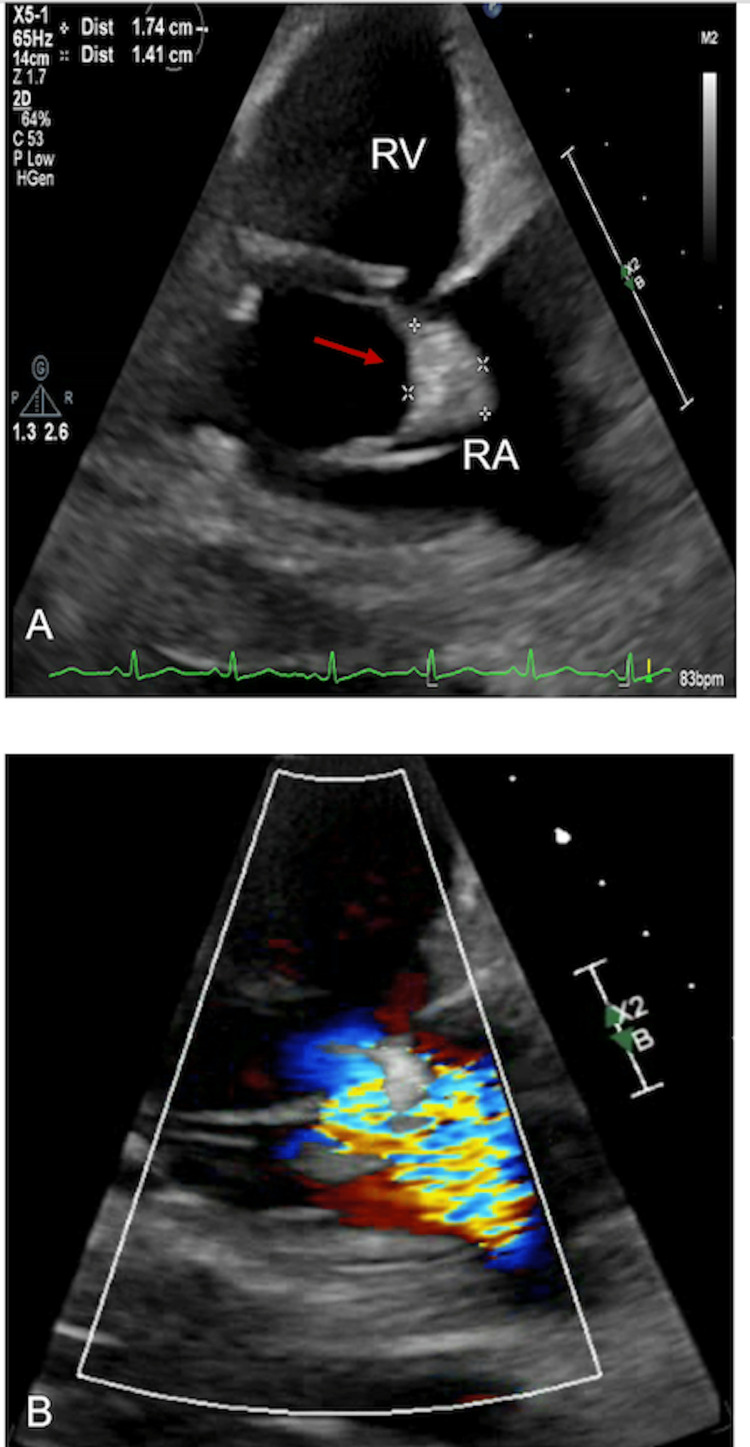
(A) 14 mm × 17 mm vegetation on the anterior leaflet of the tricuspid valve identified by the red arrow (arrow). (B) Color Doppler showing a large regurgitative jet. RV: right ventricle; RA: right atrium.
